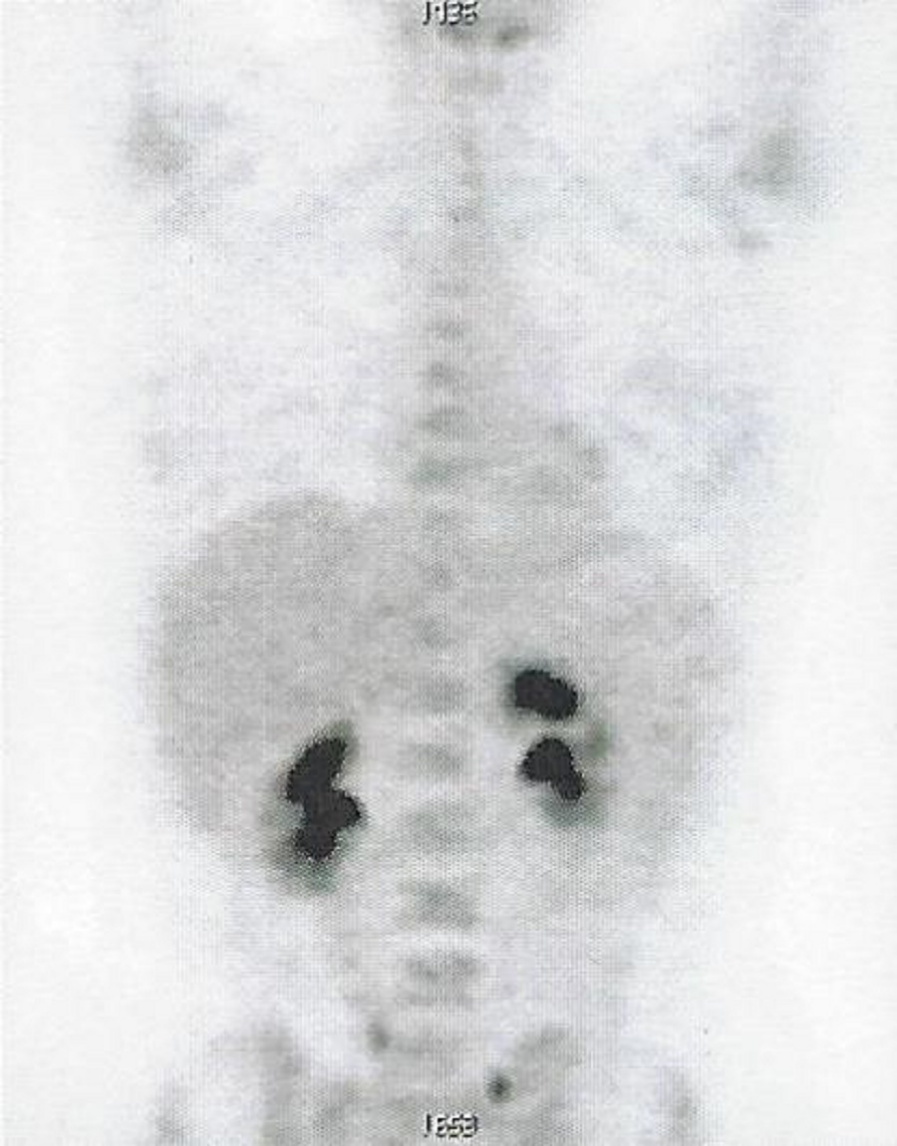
Follow-up whole body (18F)-FDG-PET-CT scan showing no pathological FDG uptake, indicative of a complete response. Abbreviations: CT, computed tomography; FDG, fluorodeoxyglucose; PET, positron emission tomography.
